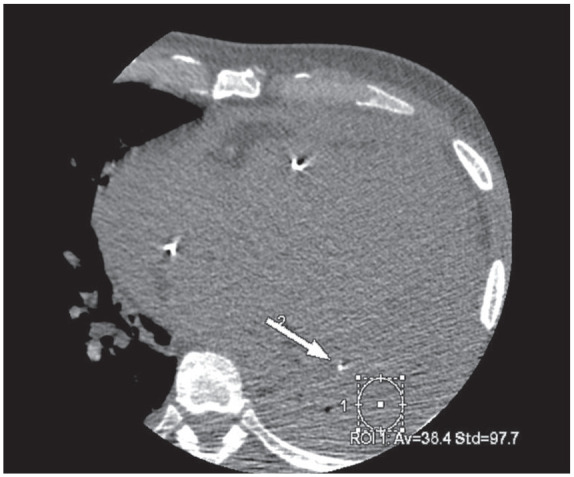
Axial view of coronary computed tomography angiography study without contrast. Arrow 2 indicates pericardial drain. Circle 1 shows the Hounsfield units in the surrounding area is 38.4, consistent with hemopericardium.
